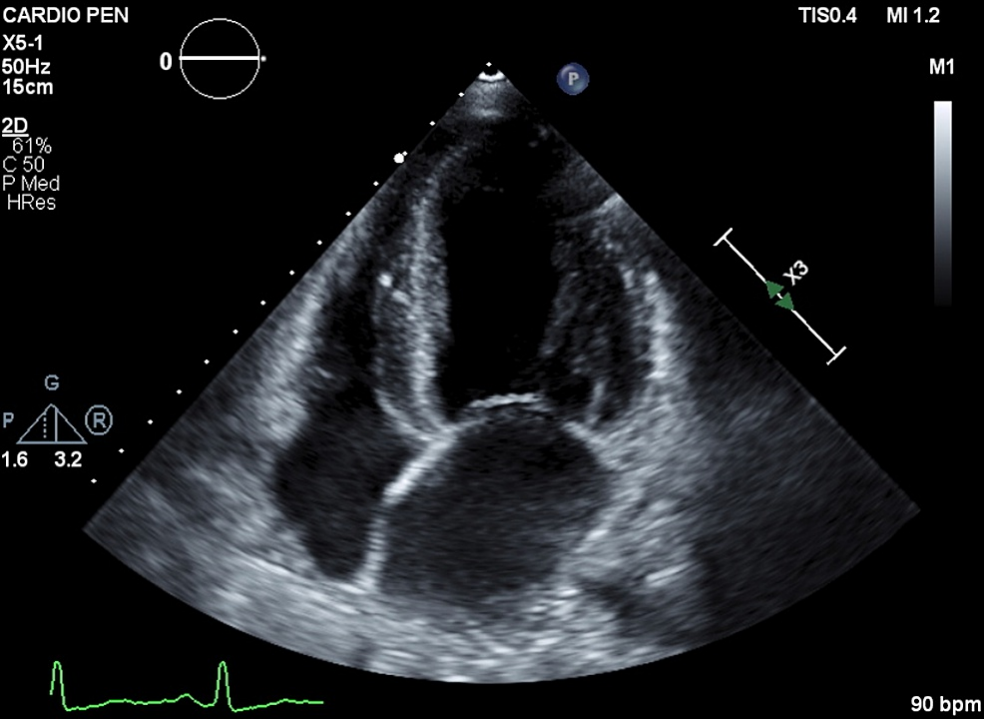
Echocardiography of the patient showing a slight left ventricular hypertrophy bpm: beats per minute
